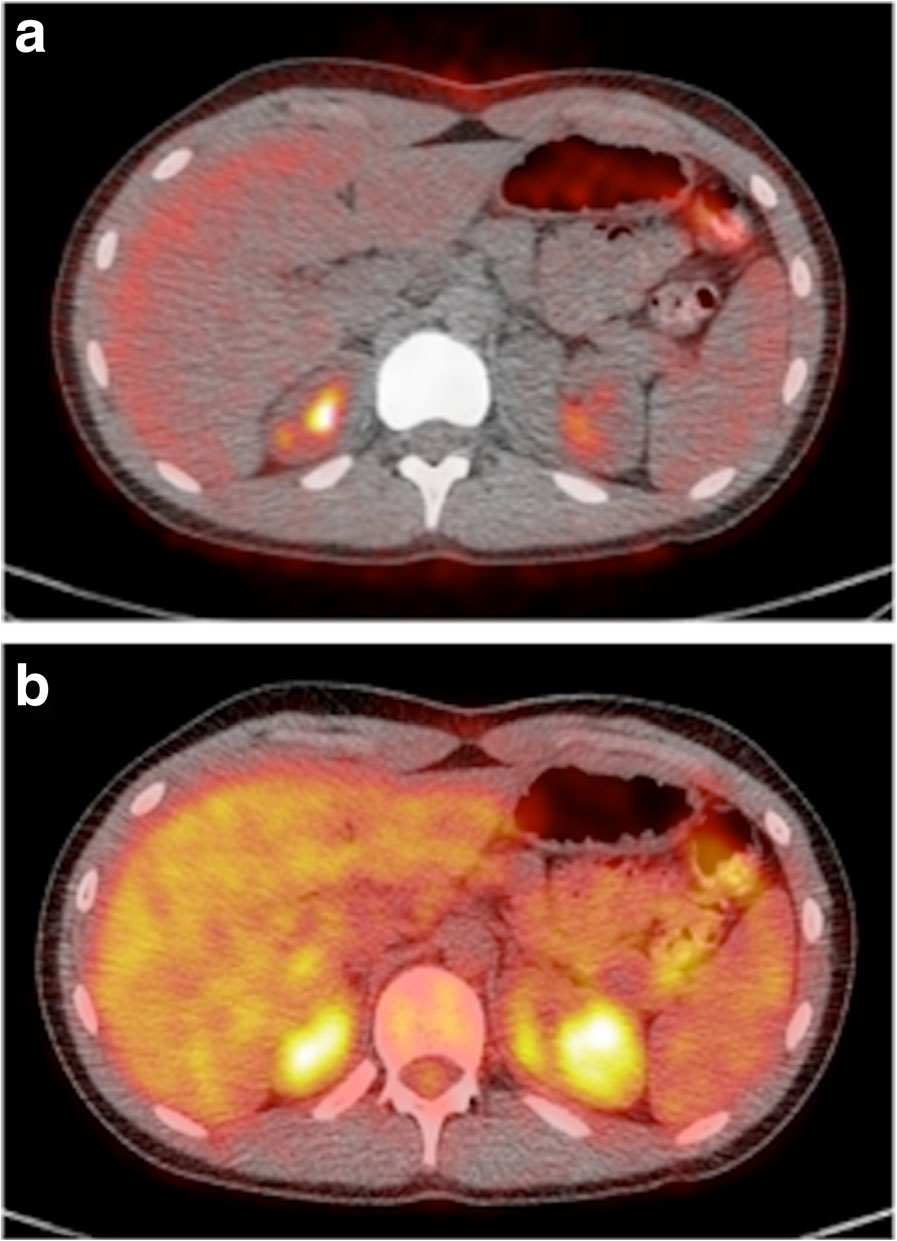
CT based attenuation correction of PET images. Low dose attenuation correction CT exams are not generally used for routine diagnostic interpretation. The AC CT accounts for differences in location/depth from which the 511 keV PET photons are emitted, and can be used to correct for differences in surrounding tissue density (HU density) and the degree to which those tissues absorb or attenuate the PET photons [5]. a shows the fused, uncorrected PET data, with increased signal at the periphery of the image and poor signal from middle of the torso and adjacent to the vertebral column. The attenuation corrected image of the same PET data (b) provides a more accurate representation of the ^18^F-FDG uptake
